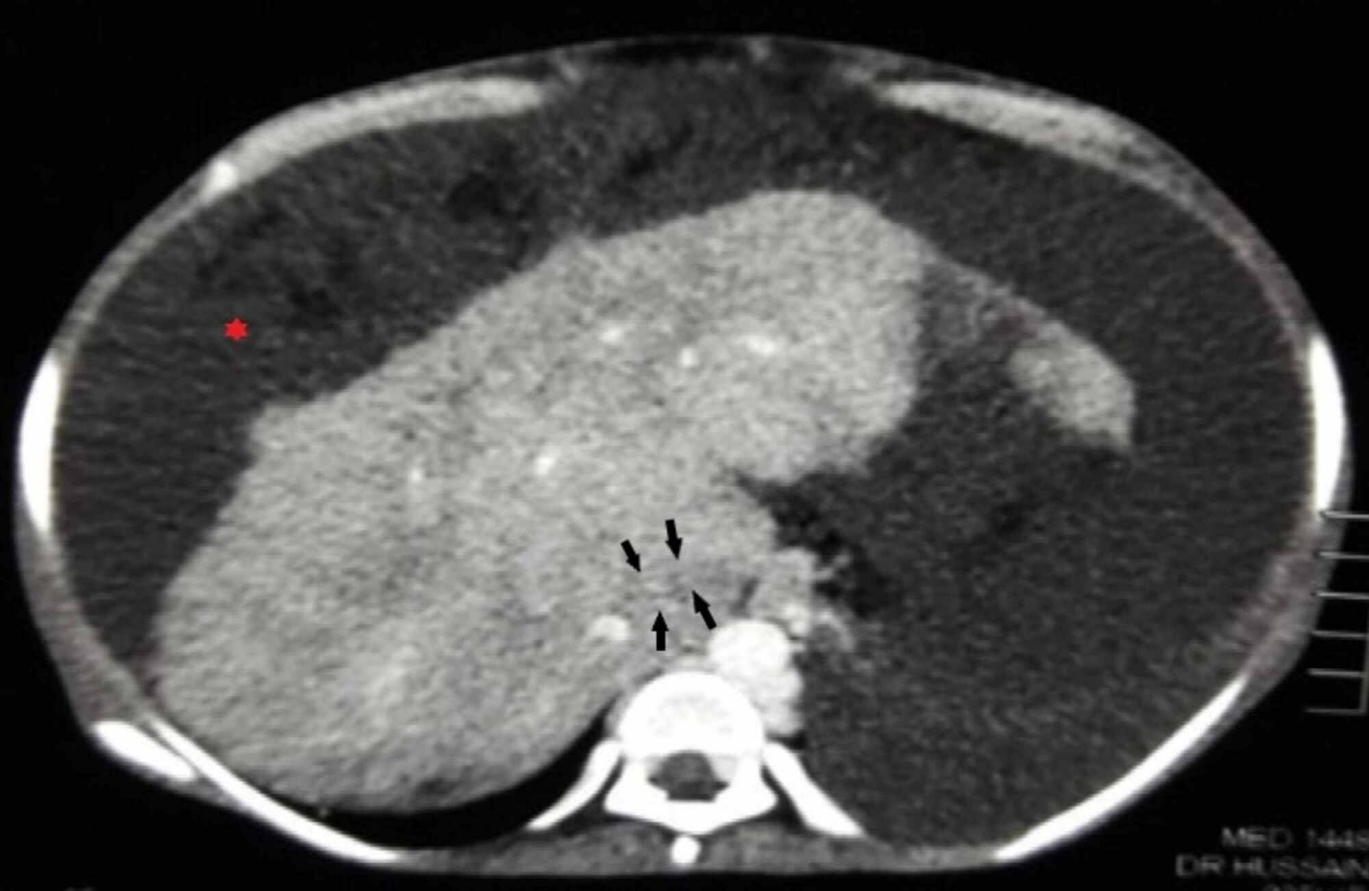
Axial contrast computed tomography (CT) obtained during venous portal phase shows non-opacification of hepatic vein (black arrow) with evidence of ascites (*).
